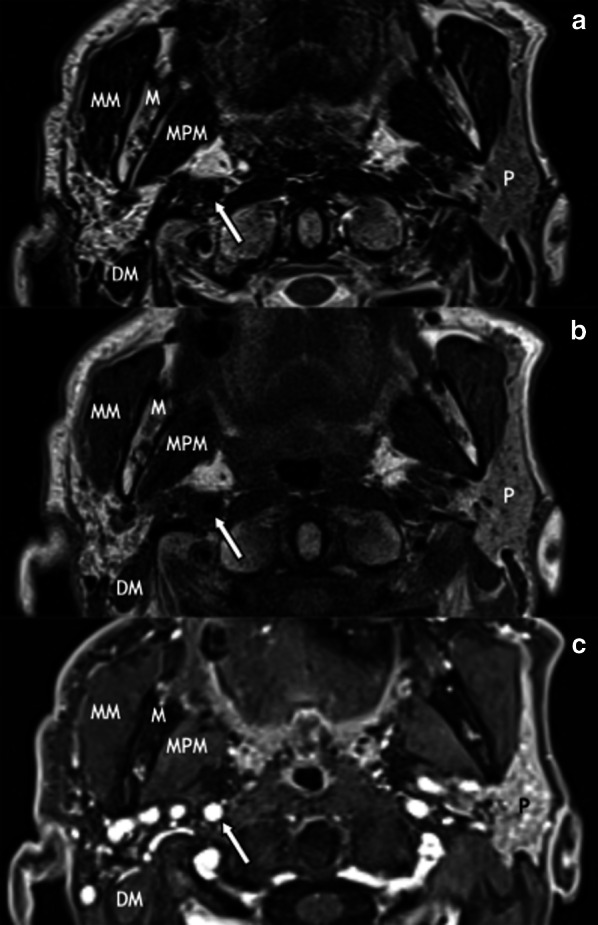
Atrophy of the right parotid gland in a 73-year-old man 3 years after ChT/RT for a carcinoma of the right inferior alveolar crest. MR images show a right parotid gland smaller than the contralateral, with high signal on axial T2-weighted (a), high signal on T1-weighted (b), and low signal on post-contrast 3D GRE fat-sat T1-weighted (c) images, due to extensive fatty infiltration of the parenchyma. P, parotid gland; M, mandible; MM, masseter muscle; MPM, medial pterygoid muscle; DM, digastric muscle; internal carotid artery (arrow)
